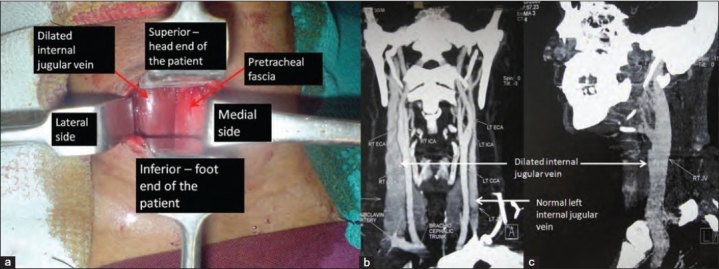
(a) Clinical photograph showing right sided anterior Smith Robinson approach of cervical spine with dilated internal jugular vein (arrow); CT venogram of the cervical spine, anteroposterior (b) and lateral (c) views shows dilated right internal jugular vein when compared to the normal left side
